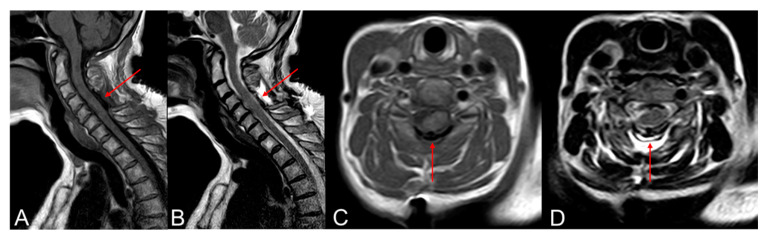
Titanium non-penetrating clips in the postoperative MRI (arrow). The use of clips does not compromise the quality of postoperative MRI images and does not show significant artifacts. T1 sagittal and axial plane (A,C). T2 sagittal and axial plane (B,D).
